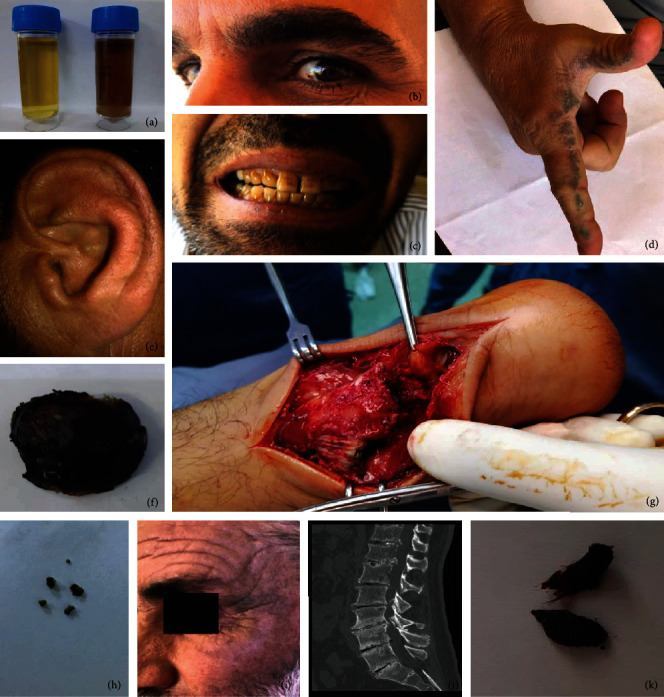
The clinical features appeared in the investigated AKU patients. (a) The urine is converted from yellow to dark brown upon overnight standing, (b) brown spot appears in the sclera of the eye, (c) dark pigmentation of the teeth, (d) blue-black papules and plaques with pitting along the line of transgradience of the thumb and index finger, (e) bluish discoloration of the external ear cartilages, (f) ochronosis of the cartilage that cover the head of the humerus bone which is removed during the shoulder joint replacement surgery, (g) Achilles tendon rupture, (h) black renal stones from AKU patient, (i) ochronosis of the face and cheeks, (j) multiple degenerative changes in the dorsal spine with narrowing and calcifications of the intervertebral disc space shown in CT scan, and (k) black colour of the ear wax.
